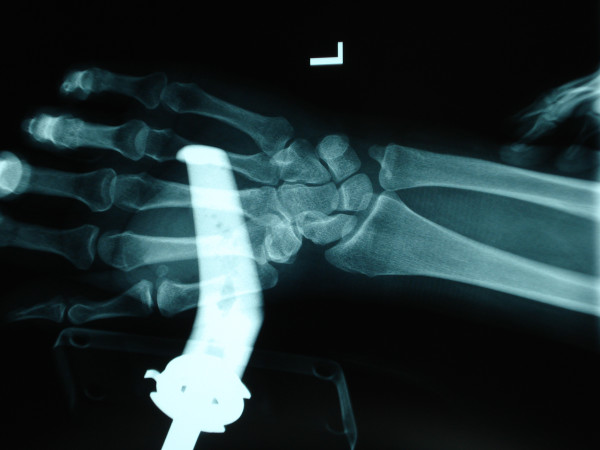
Antero-posterior radiograph of hand.
